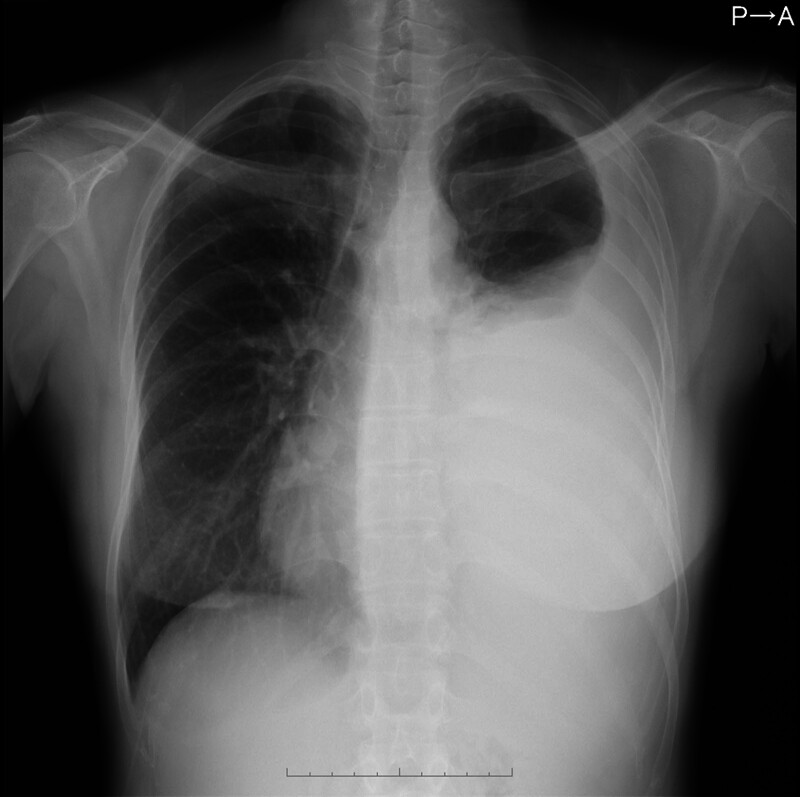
Chest radiograph showing massive left pleural effusion.
